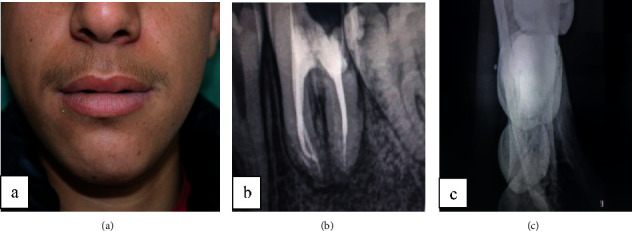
(a) Clinical healing after 4 months with extraoral swelling disappearance. (b) Periapical radiographic control after 8 months showed periapical lesion regression. (c) Occlusal radiograph showed periostitis disappearance after 4 months.
